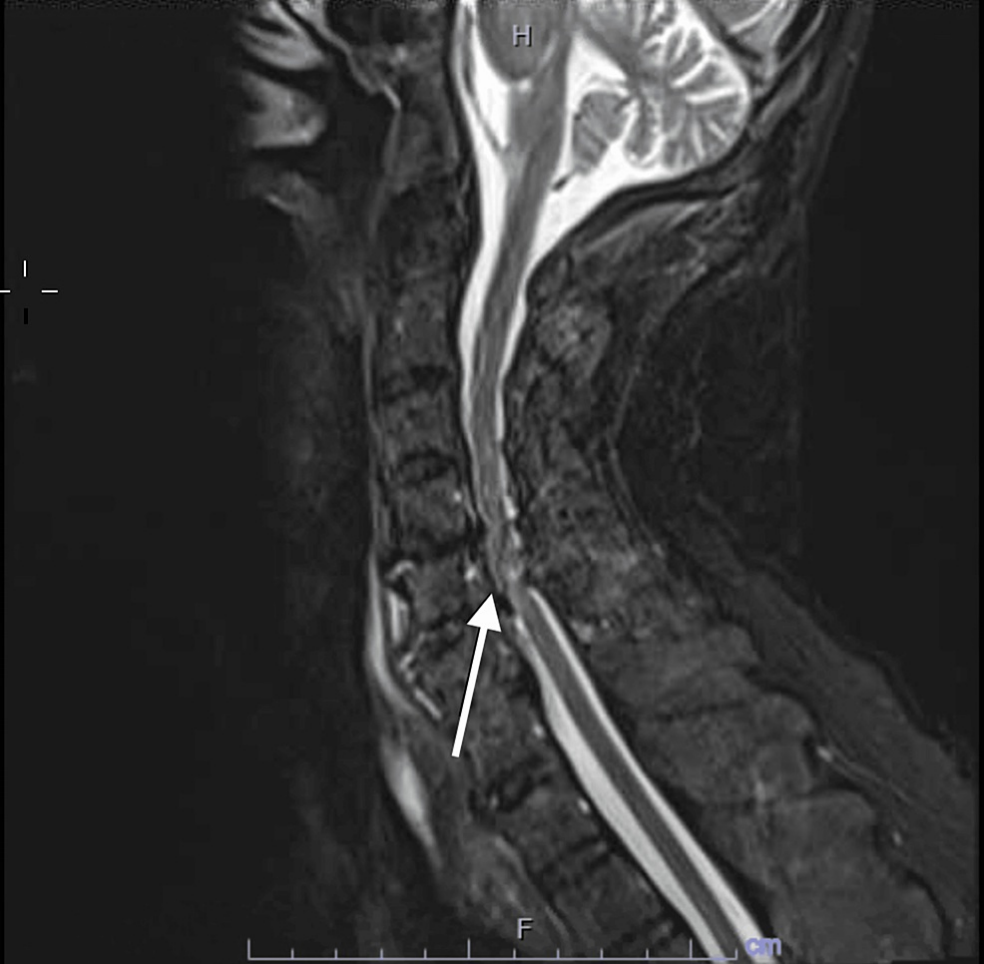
MRI Cervical Spine MRI cervical spine showing evidence of significant C5-C6 level spinal stenosis (arrow).
